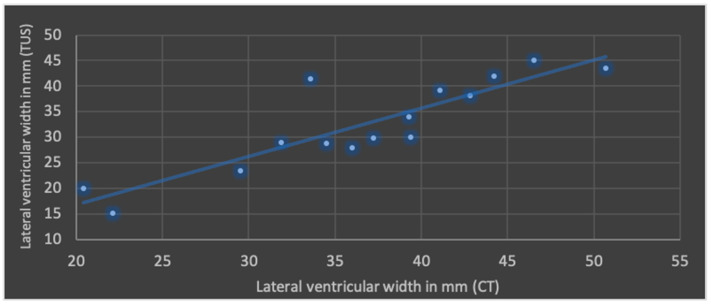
Correlation of transcranial ultrasound (TUS) and computed tomography (CT) in terms of lateral ventricular width (r = 0.88; *p* < 0.001; mean value TUS: 32.5 ± 9.0 mm; mean value CT: 36.6 ± 8.4 mm).
